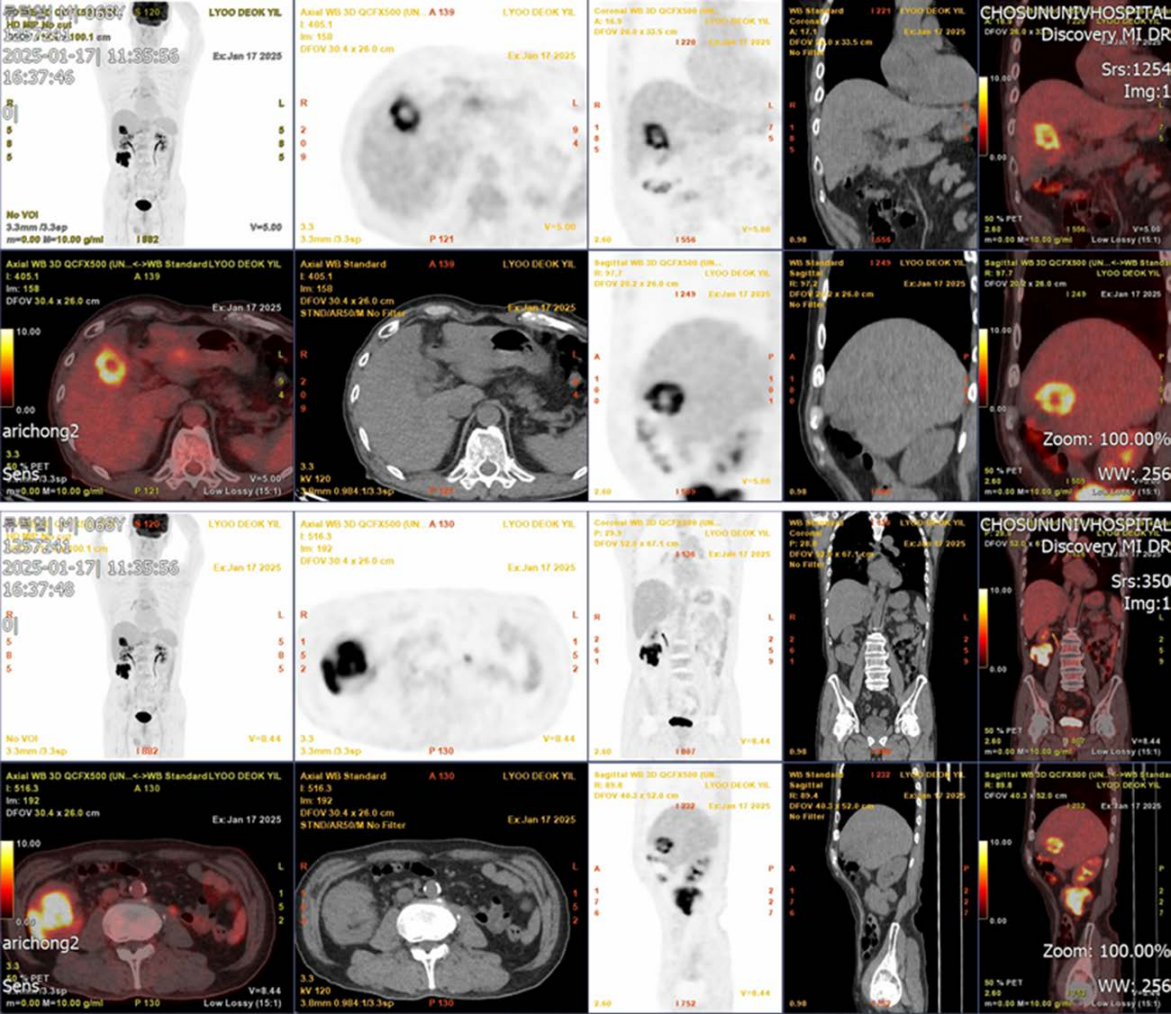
Preoperative imaging findings. F-18 FDG PET-CT shows hypermetabolism in approximately 6 cm-sized mass in the ascending colon, and in irregular mass involving the GB and adjacent liver.
